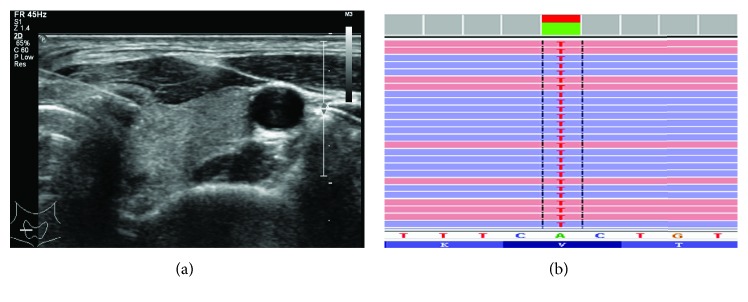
Sonogram of US-guided FNA biopsy for a 36-year-old woman with PTMC (a). NGS of the FNA sample showed a BRAF p.V600E (c.T1799A) mutation (b).
